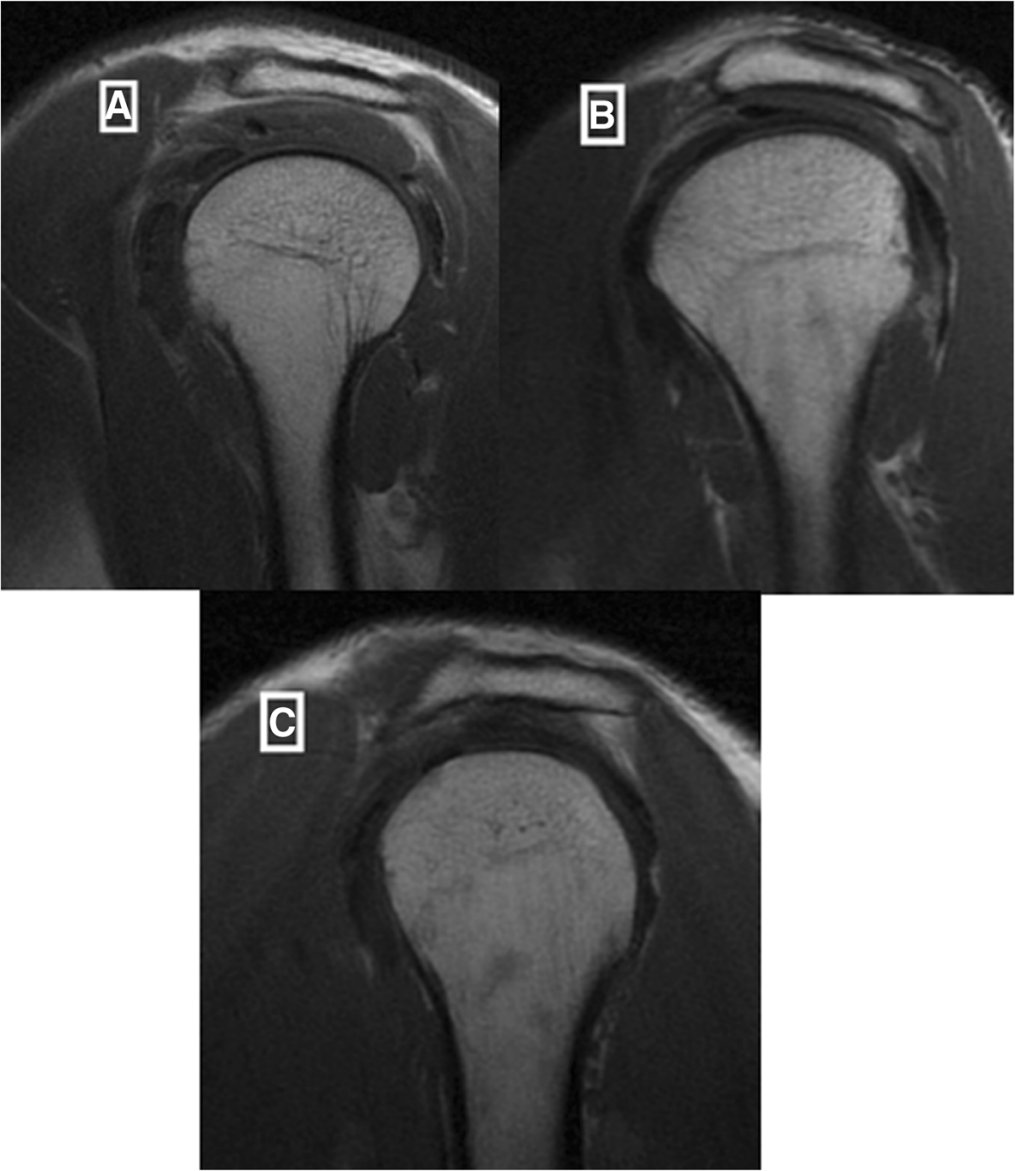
Acromial morphology in the sagittal plane in a T1-weighted sequence: flat (a), curved (b) and hooked (c)
